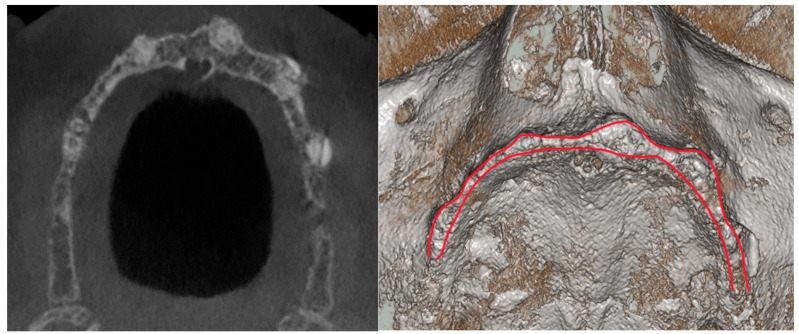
Pre-operatory CBCT. Detail of the reabsorbed maxilla.
